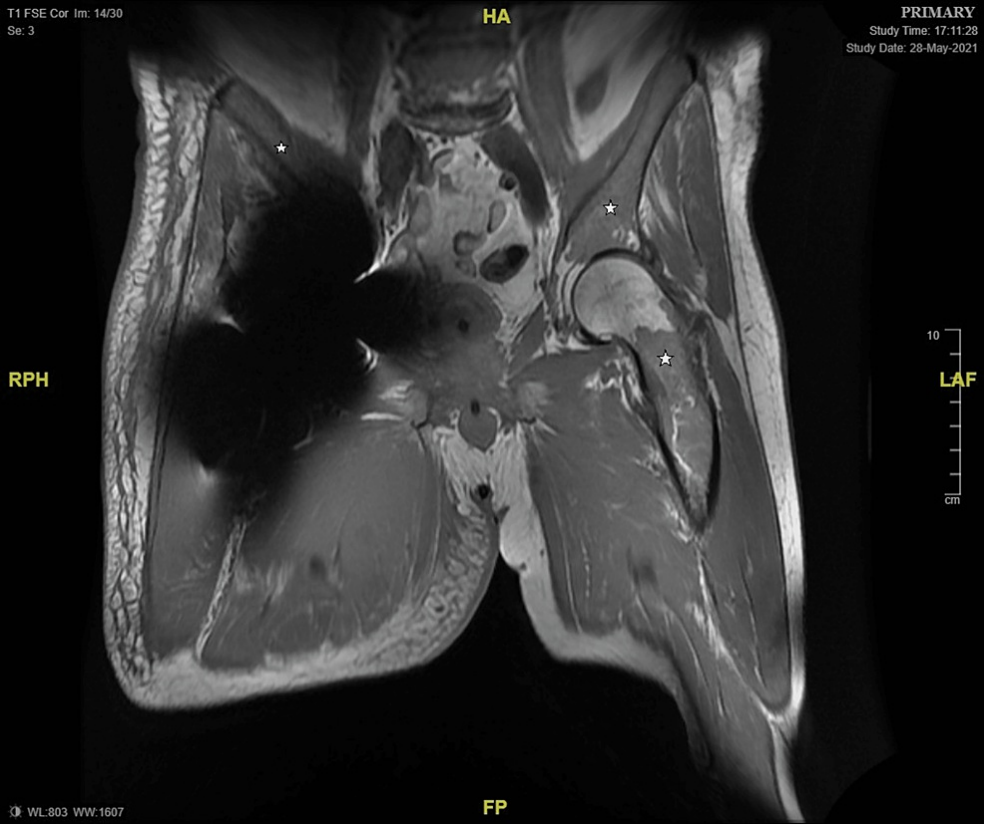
MRI hip - T1-weighted image Star: Marrow hyperplasia of pelvis and femur
